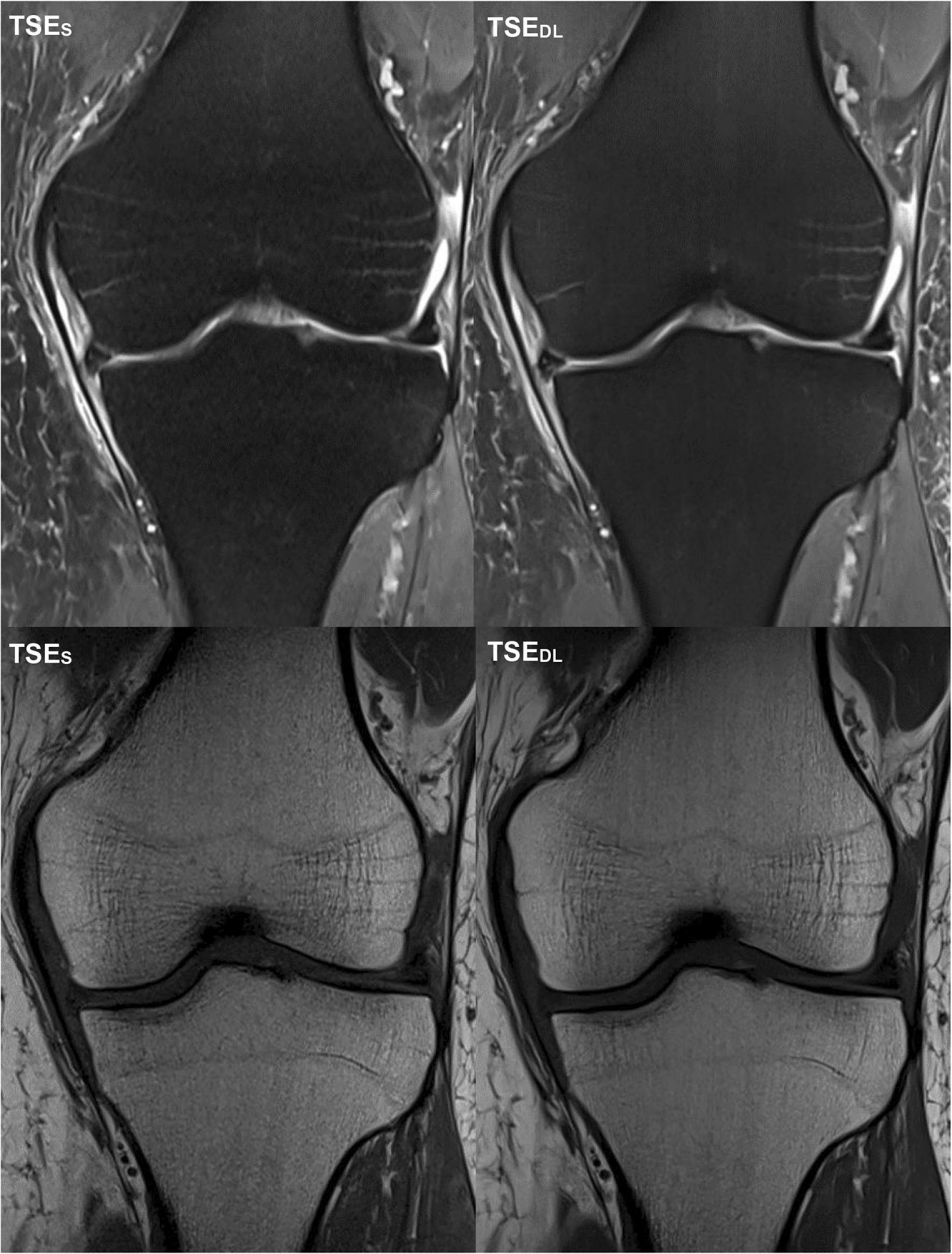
Image example of a standard and deep-learning-reconstructed PD– and T1–weighted TSE imaging of the knee at 1.5 T. This is an example of a knee MRI at 1.5 T in coronal orientation of a 59-year-old patient after partial resection of the medial meniscus. PD TSE_S_ (upper row, left) and PD TSE_DL_ (upper row, right) and T1w TSE_S_ (lower row, left) and PD TSE_DL_ (lower row, right). Comparing PD TSE (upper row) and T1w TSE (lower row), the effect of the noise reduction is more present in PD TSE_DL_ compared to PD TSE_S_ than comparing T1w TSE_DL_ compared to T1w TSE_S_
